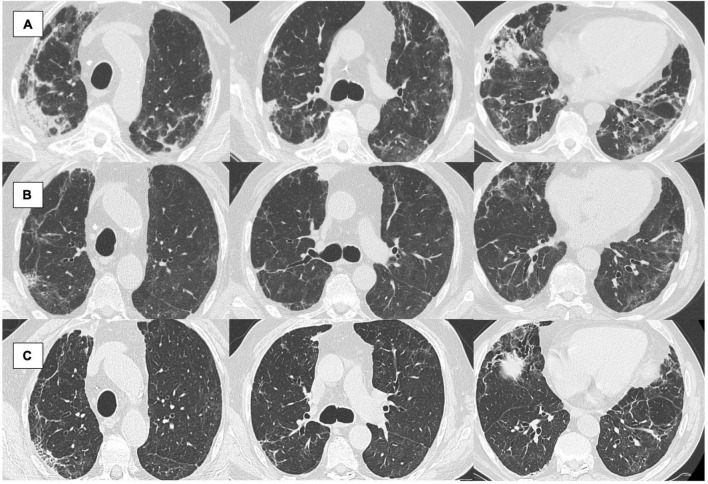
(A–C) An 84 years-old male patient followed in our post-COVID-19 clinic. (A) Computed tomography (CT) scan at 1 month from hospital admission: diffuse ground-glass opacities (GGO), also with consolidations (CO) in particular in the apical subpleural region and right basal; (B) CT at 6 months from hospital admission: ameliorated, GGO persist in the lung bases and the medium lobe; still showing interstitial thickening (IT) with bronchiectasis; (C) CT scan at 12 months from hospital admission: sclerosis of the apical subclavian regions, atelectatic thickening in the right upper lobe, diffuse bronchiectasis, and dystelectasis (evaluated through the semi-quantitative grading as follows: 0% of mean GGO extension of the five lobes; 21% of mean IT; 0% CO; 1 bronchiectasis; 1 of linear and curvilinear band opacities).
